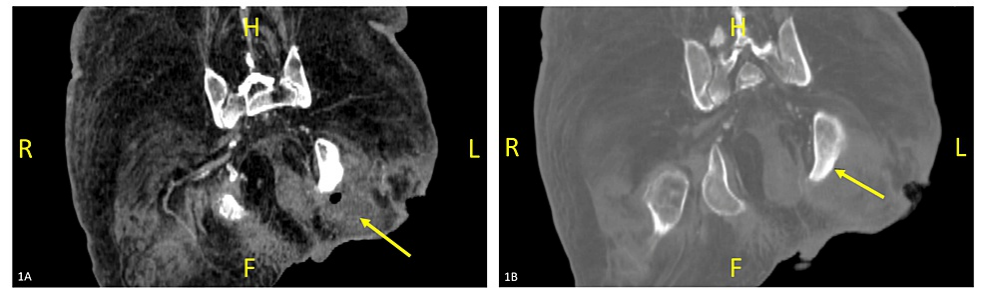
Coronal non-contact CT scan of the pelvis taken on day 20 of admission: 1A: Snapshot with arrow indicating fluid collection with gas adjacent to the left ischial bone. 1B: Snapshot with arrow indicating cortical loss with osteolysis of the left ischial bone, suggestive of osteomyelitis H: head, F: foot, R: right, L: left
